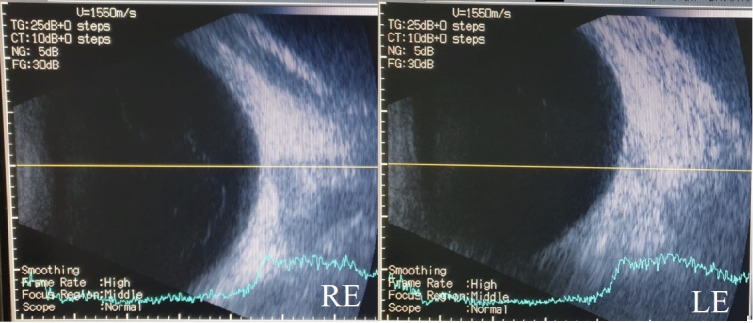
Ocular Ultrasonography (RE-Right eye; LE- Left eye)
